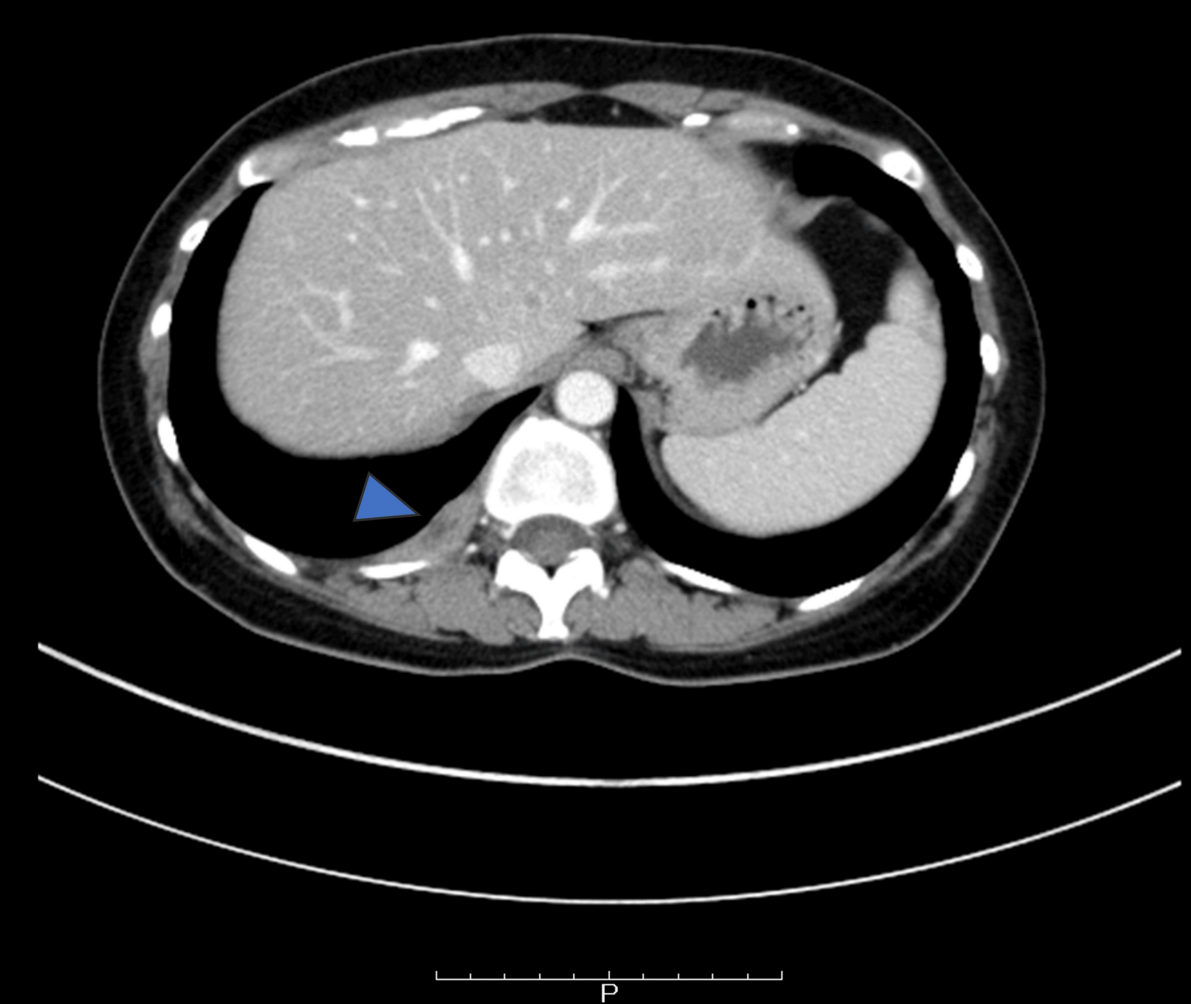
CT image at the time of transfer to our hospital showing malignant pleural effusion (indicated by arrow).
